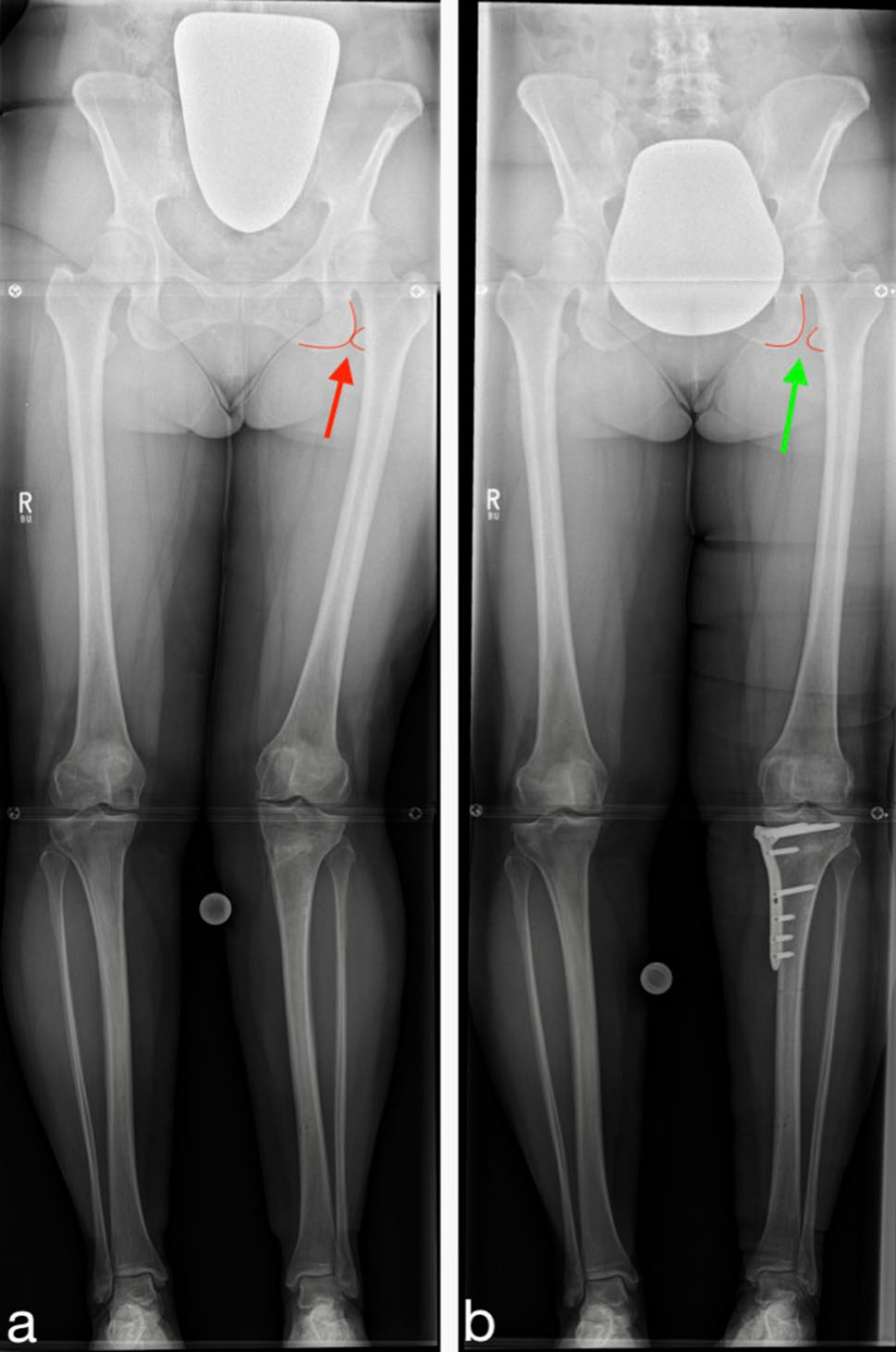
Radiographic demonstration of a patient who presented with new-onset symptomatic disabling hip pain on the left side after having undergone a high tibial valgisation osteotomy on due to medial knee gonalgia. a Over-correction with reverse obliquity of the joint line and a merely obliterated ischiofemoral space (arrow). b The situation after a closing wedge high tibial osteotomy and restoration of the ischiofemoral space 6 months postoperatively, the patient was pain free
